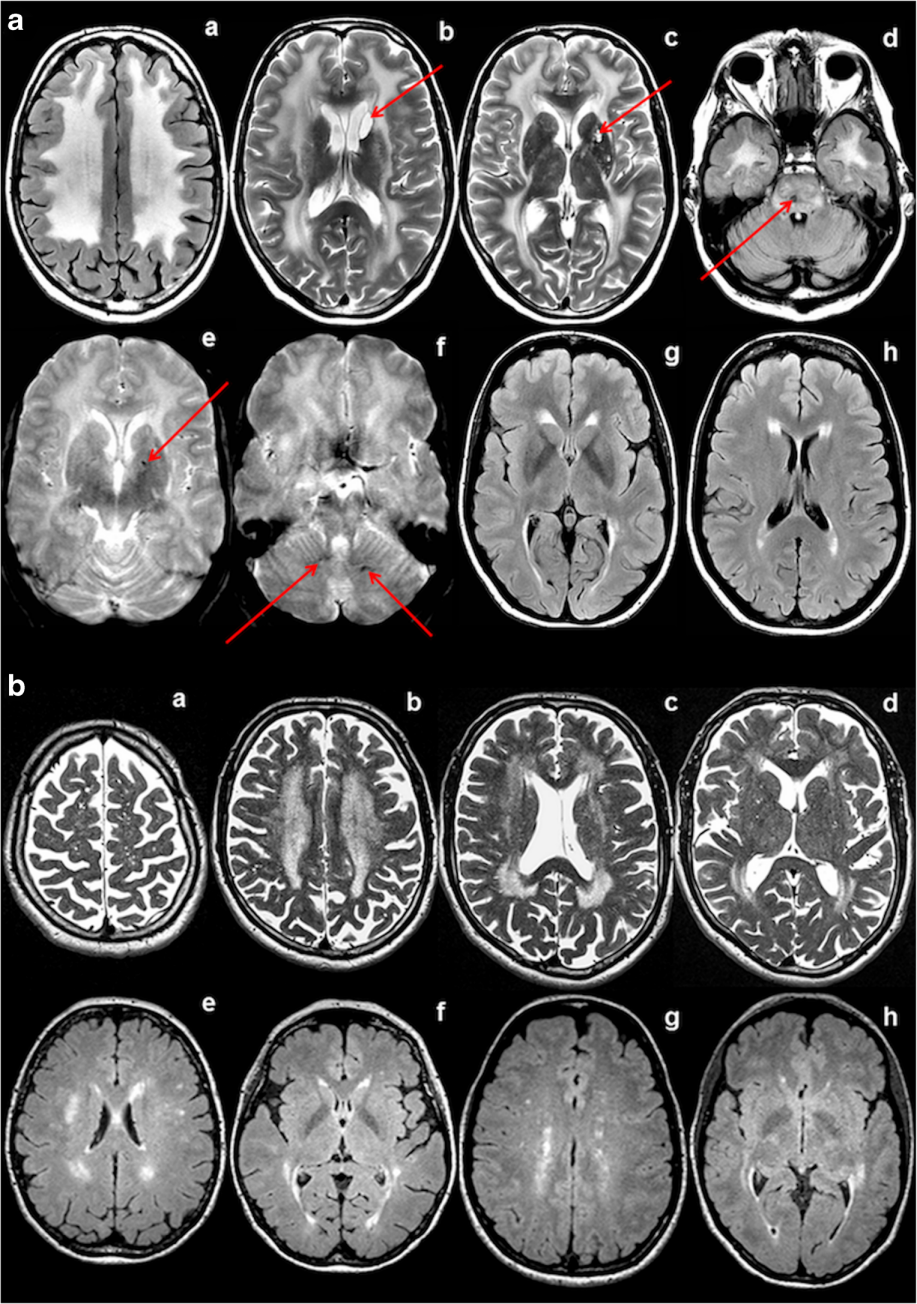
MRI. a Family A, (a–f) individual A:II-1 at age 57 years; (g, h) individual A:III-2 at age 31 years. The FLAIR (a, d) and T2-weighted (b, c) images in the older patient show virtually diffuse cerebral white matter signal abnormalities, including also the external and extreme capsules (c) and the anterior temporal white matter (d). Lacunar infarcts are seen in the head of the caudate nucleus on the left (arrow in b), left putamen (arrow in c), and pons (arrow in d). Numerous small areas of abnormal signal are seen in the basal ganglia and thalami (c) and the pons (d). Gradient echo images show microbleeds in the basal nuclei and dentate nucleus (arrows in e and f). The FLAIR (g, h) images in the younger patient show a thin periventricular rim as well as a few larger areas of abnormal signal in the deep cerebral white matter. b Family B, (a–d) individual B:III-10 at age 68 years (e, f); individual B:III-2 at age 56 years and (g, h) individual B:IV-10 at 43 years. In the oldest patient, the T2-weighted images show extensive and confluent periventricular and deep cerebral white matter abnormalities (a–d), as well as numerous enlarged perivascular spaces in the white matter (a–c) and basal nuclei (d). There is a mild generalized atrophy. The FLAIR images in the younger patients (e–h) show a thin periventricular rim as well as multifocal small and lager larger areas of abnormal signal in the deep cerebral white matter
